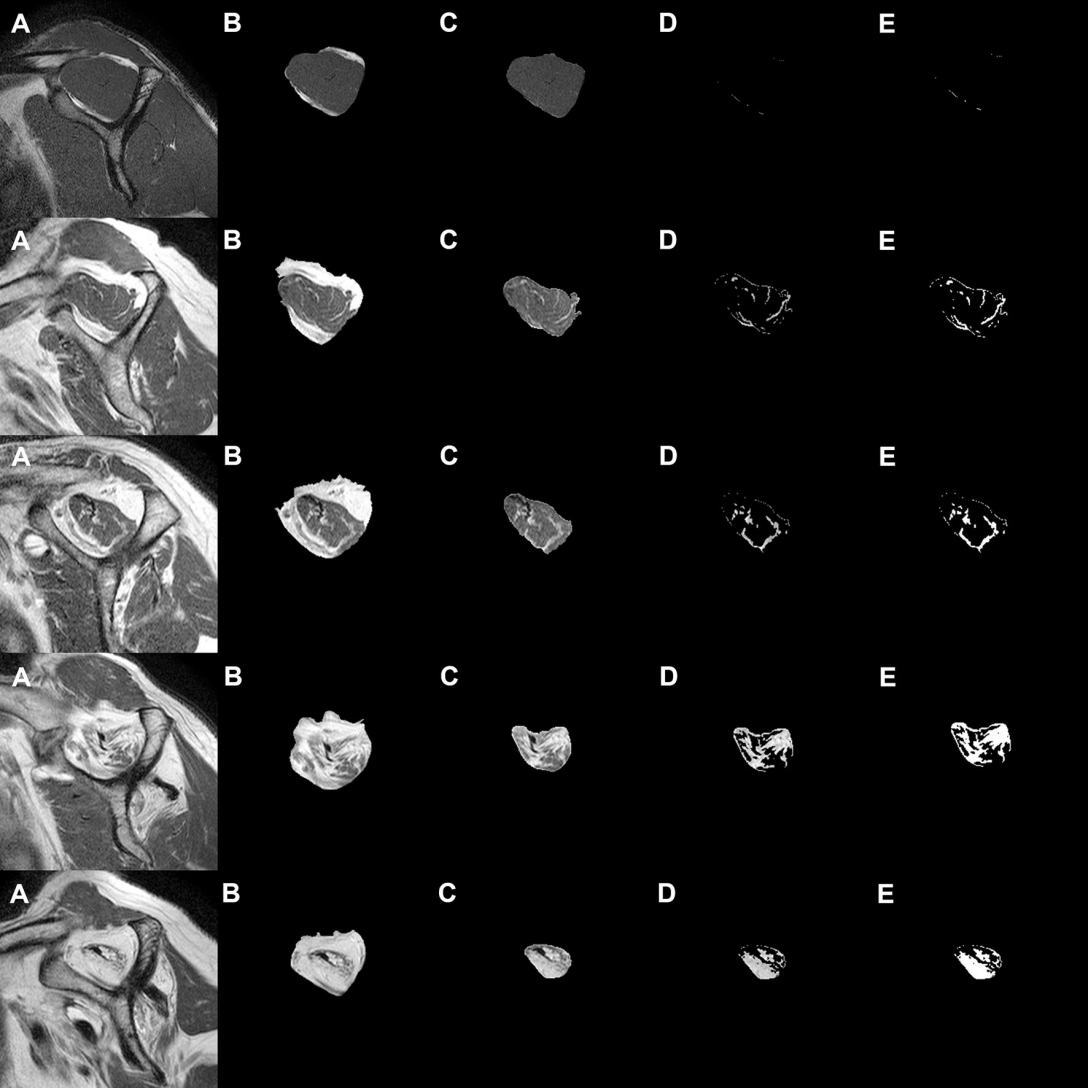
Image analysis steps of the proposed method for supraspinatus muscle segmentation by CNN and the fatty infiltration analysis by Otsu thresholding: (A) original MR image; (B) supraspinatus fossa segmentation by CNN; (C) supraspinatus muscle segmentation by CNN; (D) Otsu thresholding fatty infiltration image from the area of supraspinatus muscle; and (E) post processed final binary image. This includes black (supraspinatus muscle) and white (fatty infiltration) conversions of Otsu thresholding fatty infiltration image.
